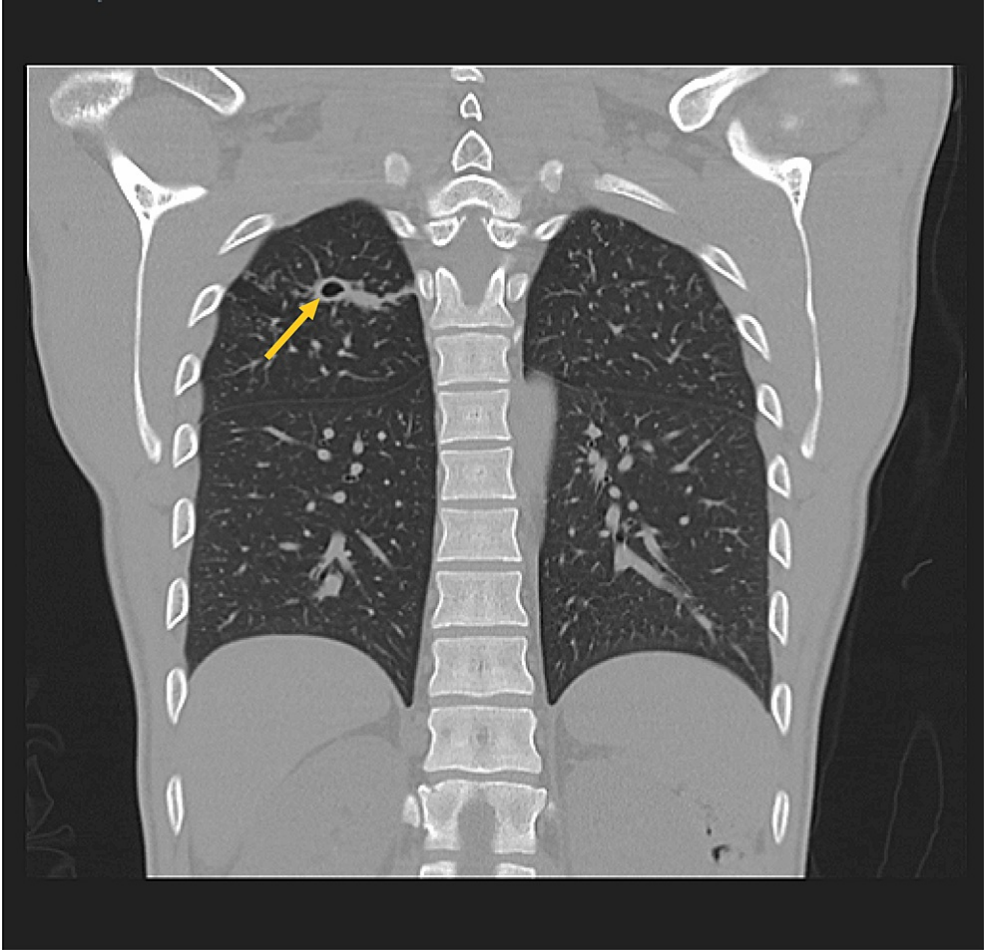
Representative coronal computed tomographic (CT) image of the chest demonstrates right upper lobe cavitary lesion compatible with infectious causes including mycobacterial and fungal etiologies.
